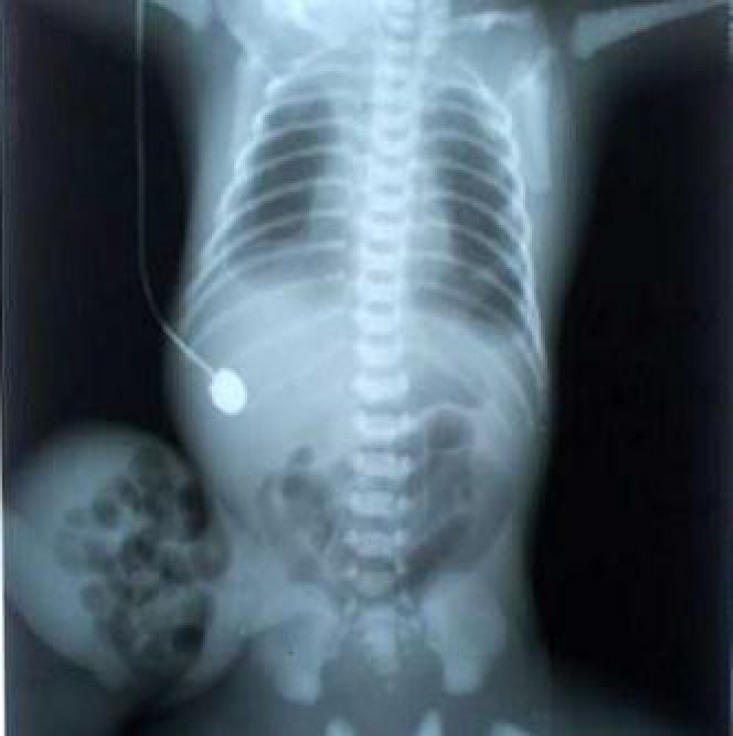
X-ray showing intestine as the content of the sac
